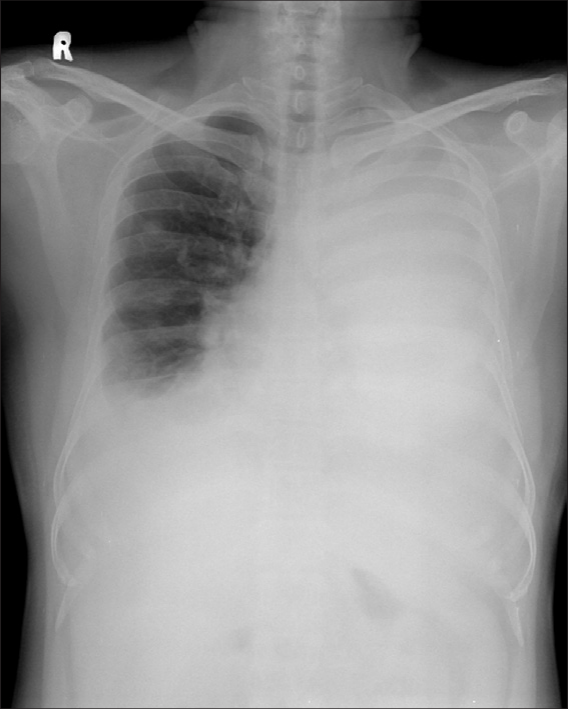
Chest radiograph showing bilateral pleural effusion, massive on left side
